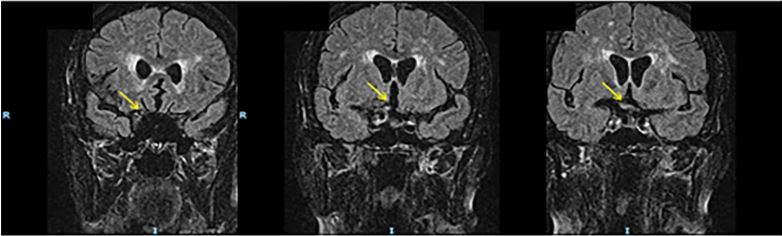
Coronal FLAIR (fluid-attenuated inversion recovery) demonstrating optic nerve compression (yellow arrow).
